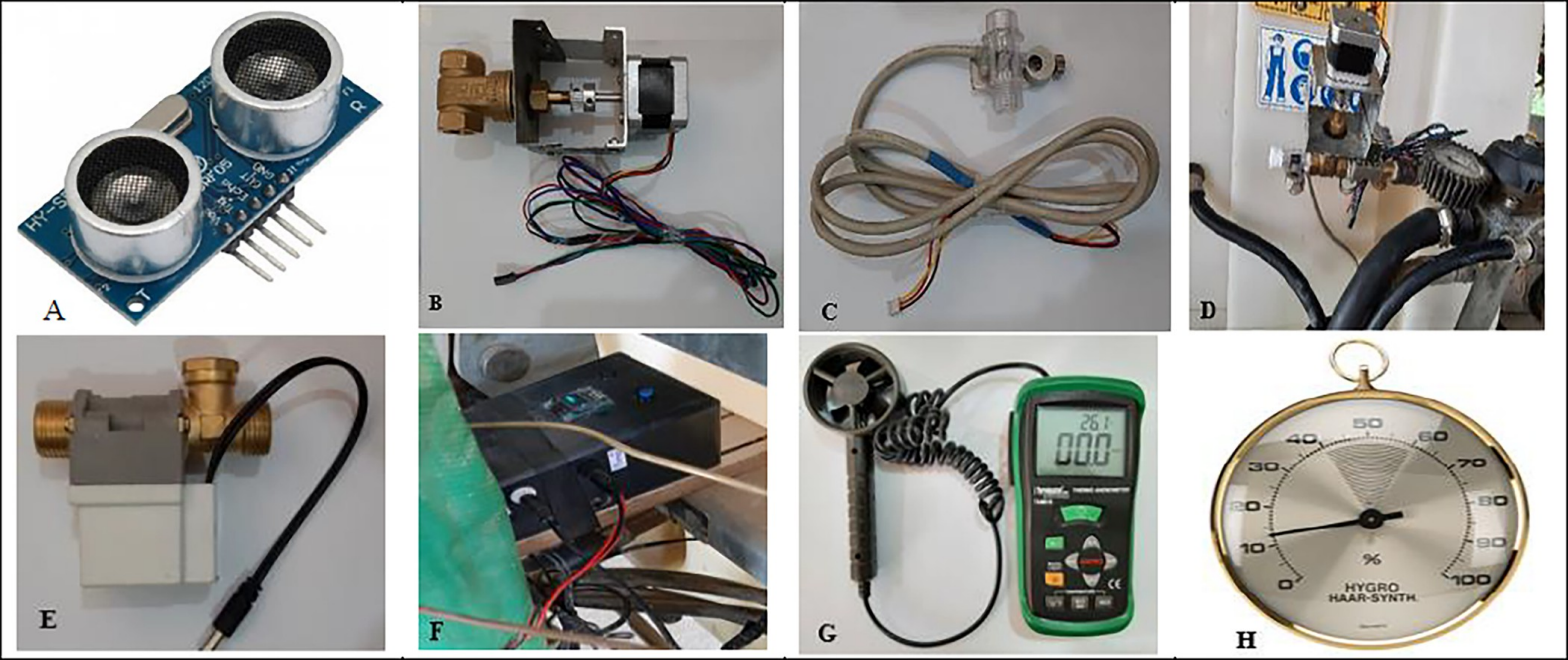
A: Ultrasonic sensor B: Variable rate valve C: Flowmeter (YF-S201C Water flow sensor) D: Installing a variable rate valve and flowmeter on the sprayer E: ON and OFF solenoid valve (electric solenoid valve 12v 24V 220V) F: Installing the electronic board on the sprayer G: Anemometer and thermometer H: Hygrometer.
